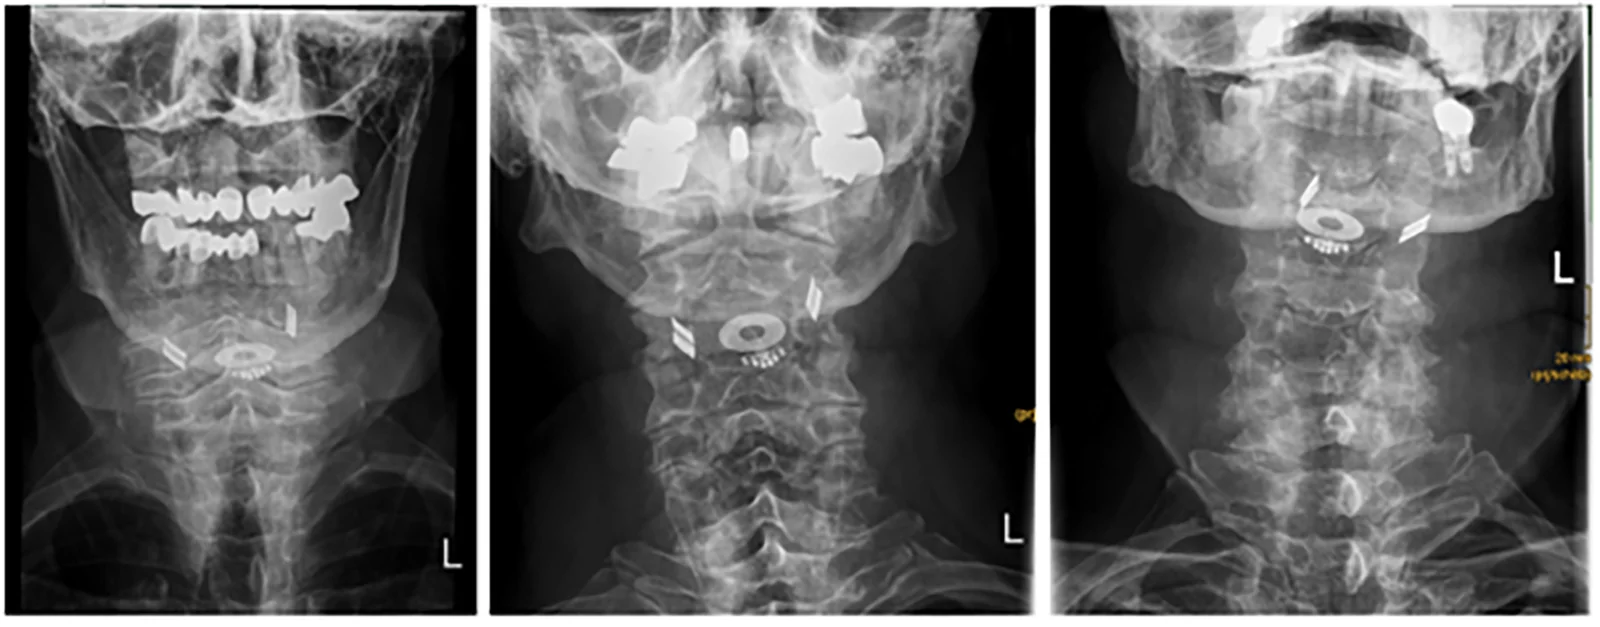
x-ray (a.p.). Asymmetrical alignment of bilateral paddle electrodes in 3 patients.
